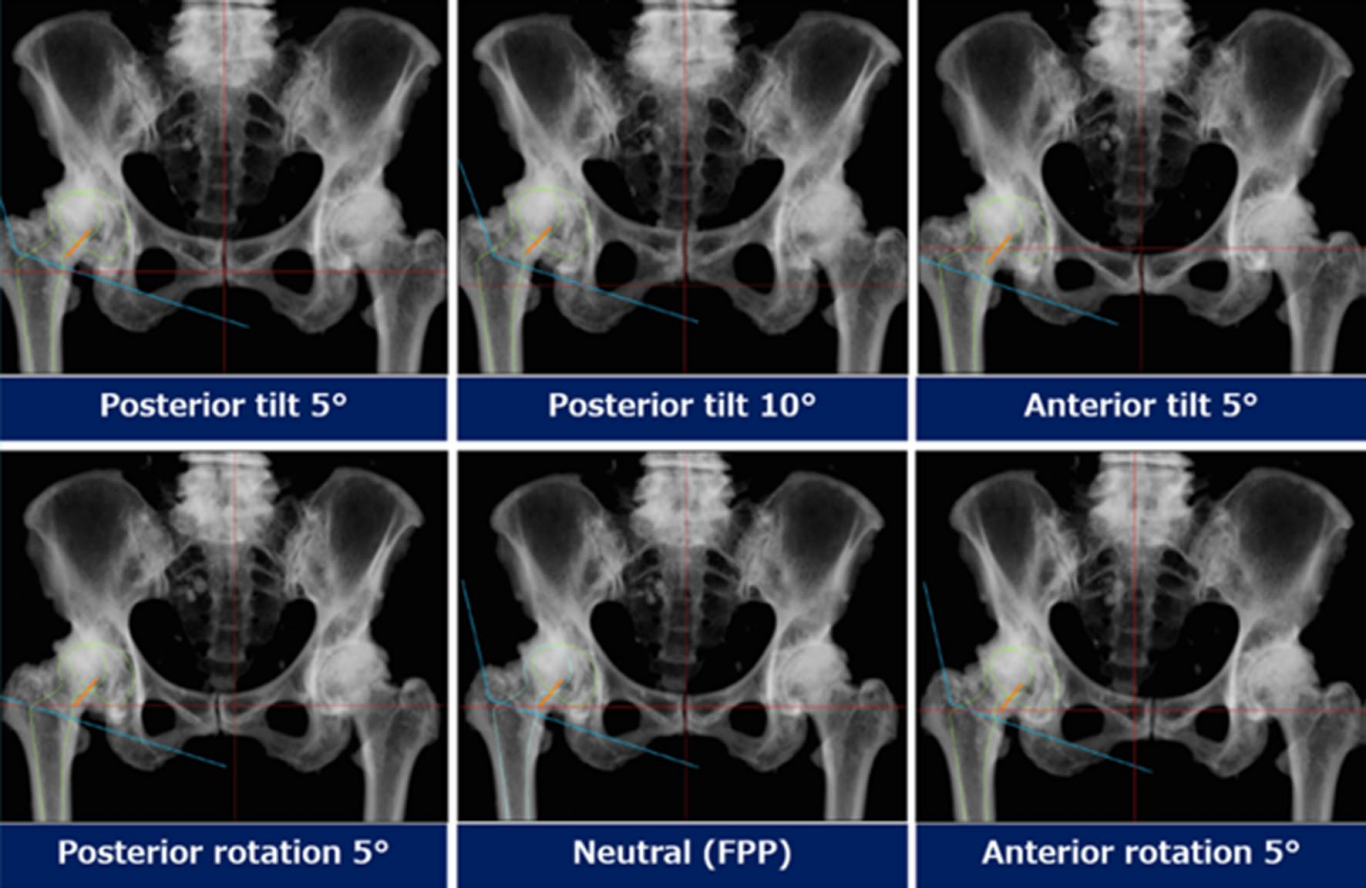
Several patterns of digitally reconstructed radiographs of the pelvis include a pelvis in the functional pelvic plane (FPP) and pelvises that changed pelvic tilt or pelvic rotation from the FPP. The pelvic position is adjusted based on these images just before surgery.
